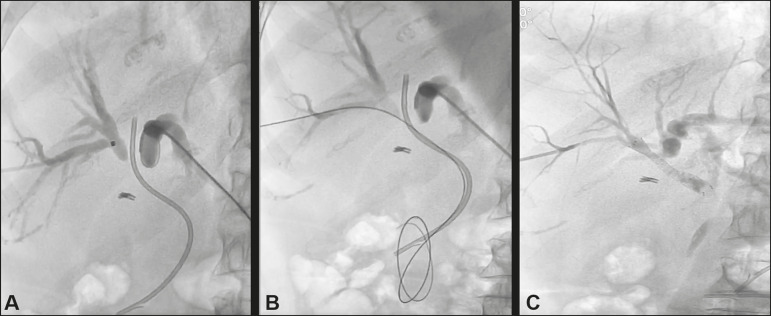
A 60-year-old female patient with Bismuth type IV cholangiocarcinoma underwent an endoscopic procedure for the implant of a 7 Fr plastic biliary stent. At 10 weeks after the procedure, her jaundice worsened. Computed tomography of the abdomen showed marked dilatation of the intrahepatic biliary tract due to improper positioning of the biliary stent. Percutaneous drainage and bilateral cholangiography (A) showed the failing plastic biliary stent. The stent was dislodged with a diagnostic catheter (B) and a new 6 × 60 mm biliary stent was placed in the right pre-papillary biliary tract (C). It was not possible to resolve the stenosis in the left biliary tract, and unilateral drainage was therefore performed. Her bilirubin levels normalized by day 30.
